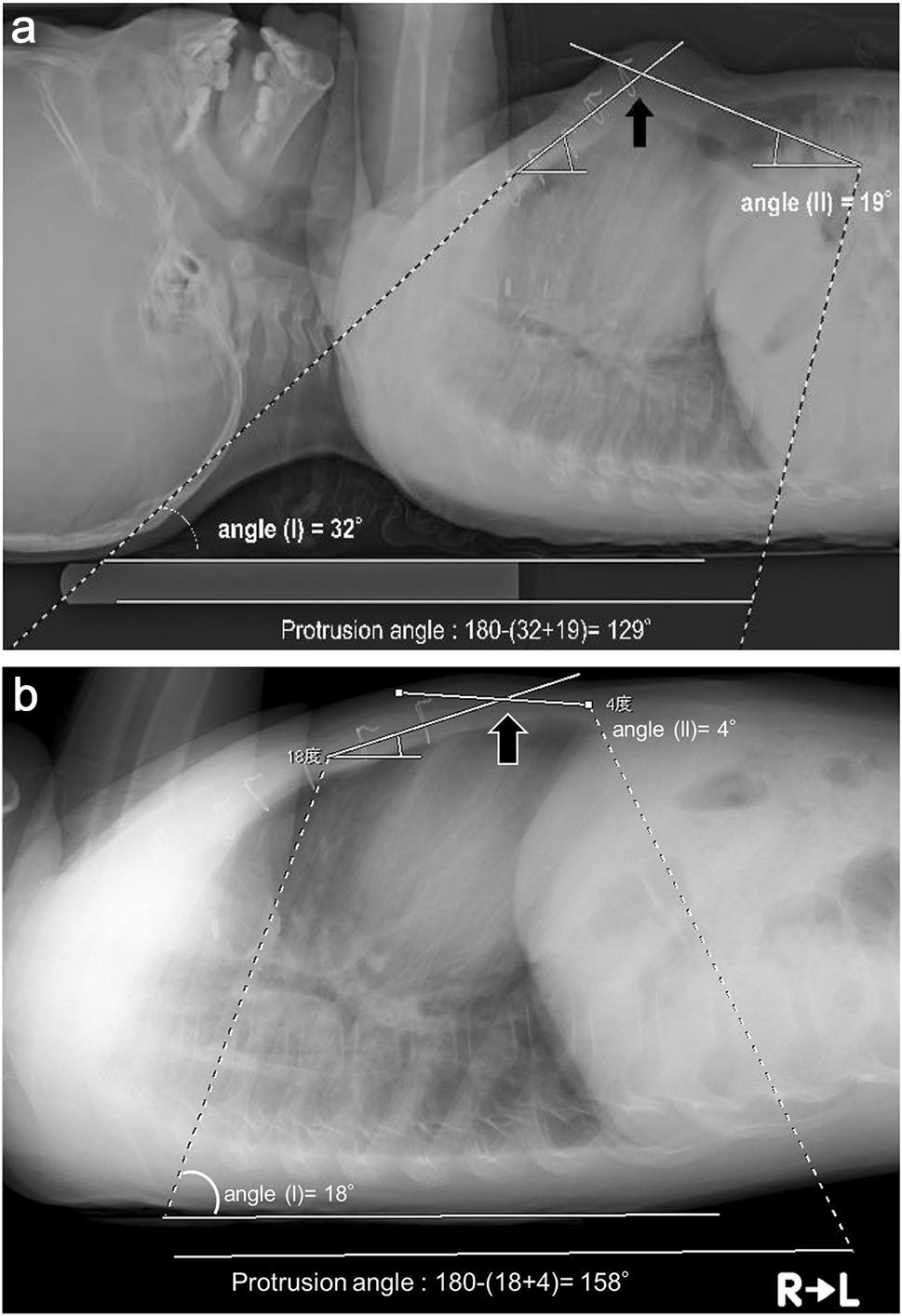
Measurement of the protrusion angle on chest X-ray. The protrusion angle (°) was then defined as 180° minus the sum of angle (I) and angle (II). Angle (I): the angle between the longitudinal axis of the sternum and the dorsal horizontal line of the CXR table. Angle (II): the angle between the descending line from the prominent point of the sternum to the anterior insertion of the diaphragm and the horizontal table line. The solid arrow (➡) indicates the protrusion angle. a 129° at the initiation of brace therapy. b 158° (+29°) after 66 months of brace therapy (same case as in Fig. 1b)
